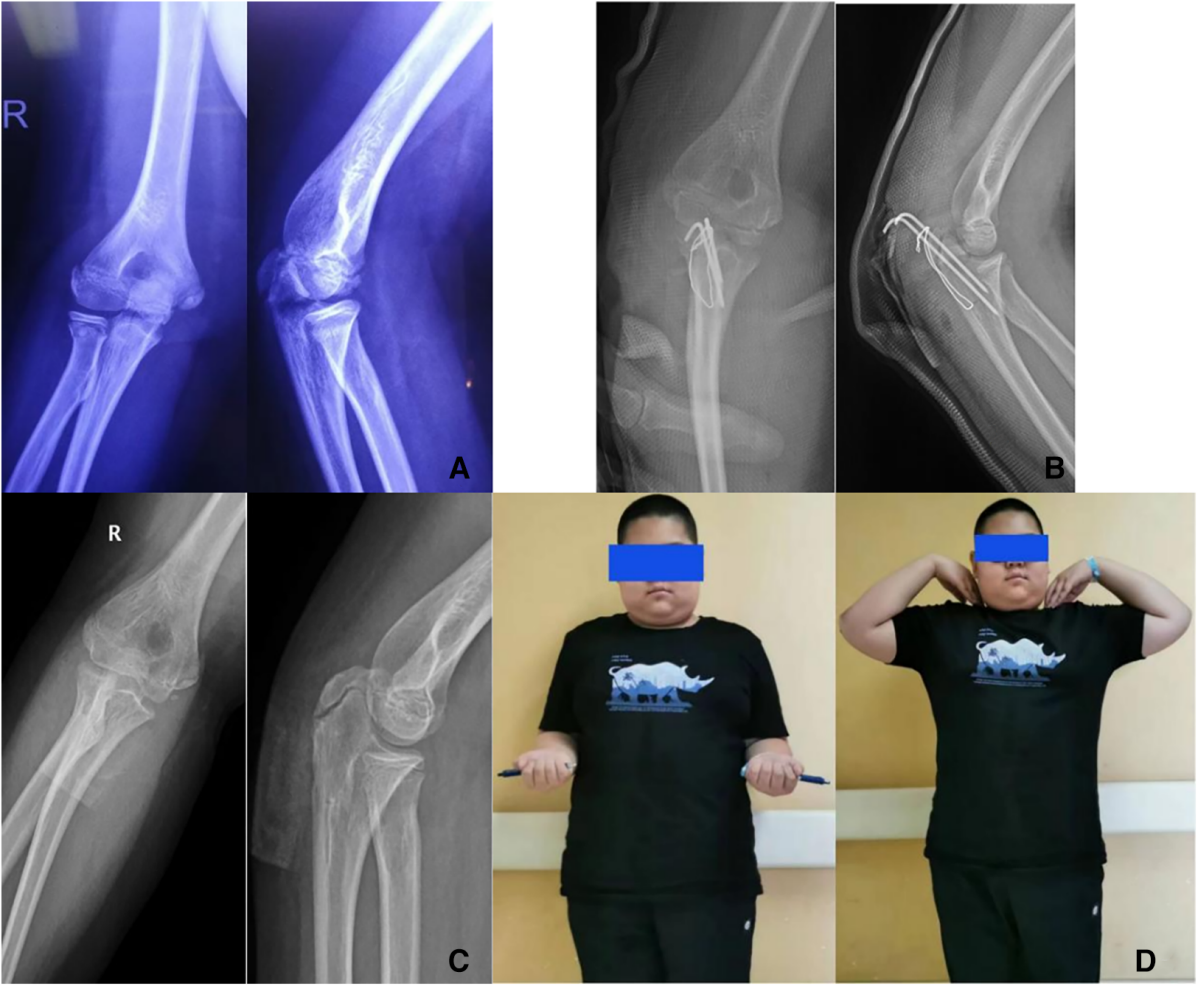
(A) Preoperative images; Anteroposterior (AP) and lateral radiographs in a 12-year-old boy with an olecranon fracture. (B) Postoperative images from the 1-day follow-up and AP and lateral radiographs in the same patient treated with open reduction and TBW fixation. (C) Follow-up images obtained at 6 months after surgery in the same patient. (D) Functional outcomes in the same patient at 6 months after surgery. Before removing the TBW screw, we recorded the patient's elbow range of motion in flexion, extension, pronation, and supination, forearm length, and QuickDASH score.
